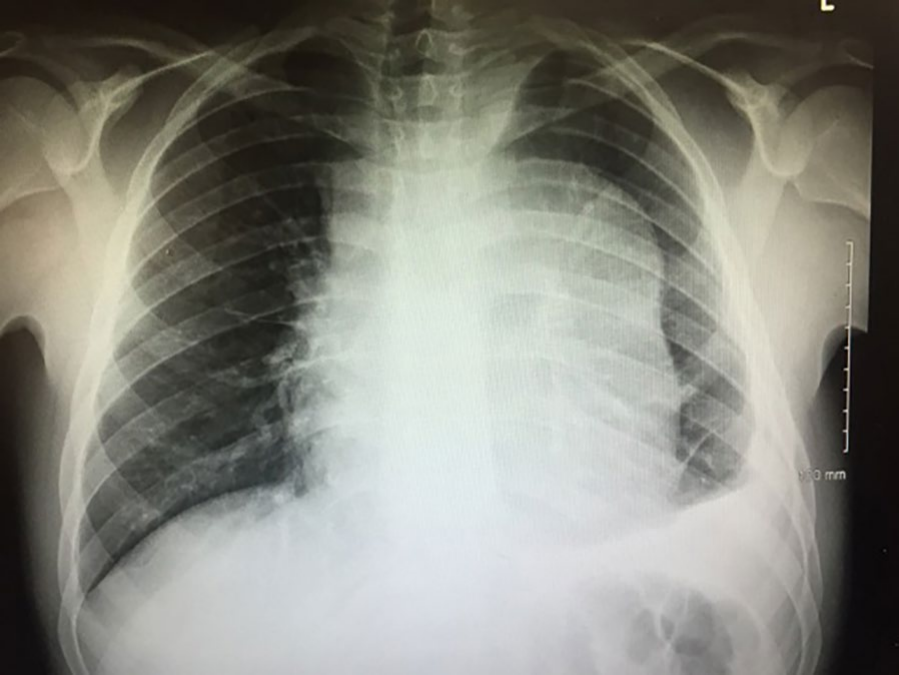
Chest radiograph, Case 2. This AP chest radiograph exhibits a widened mediastinum, suggestive of a mediastinal mass
